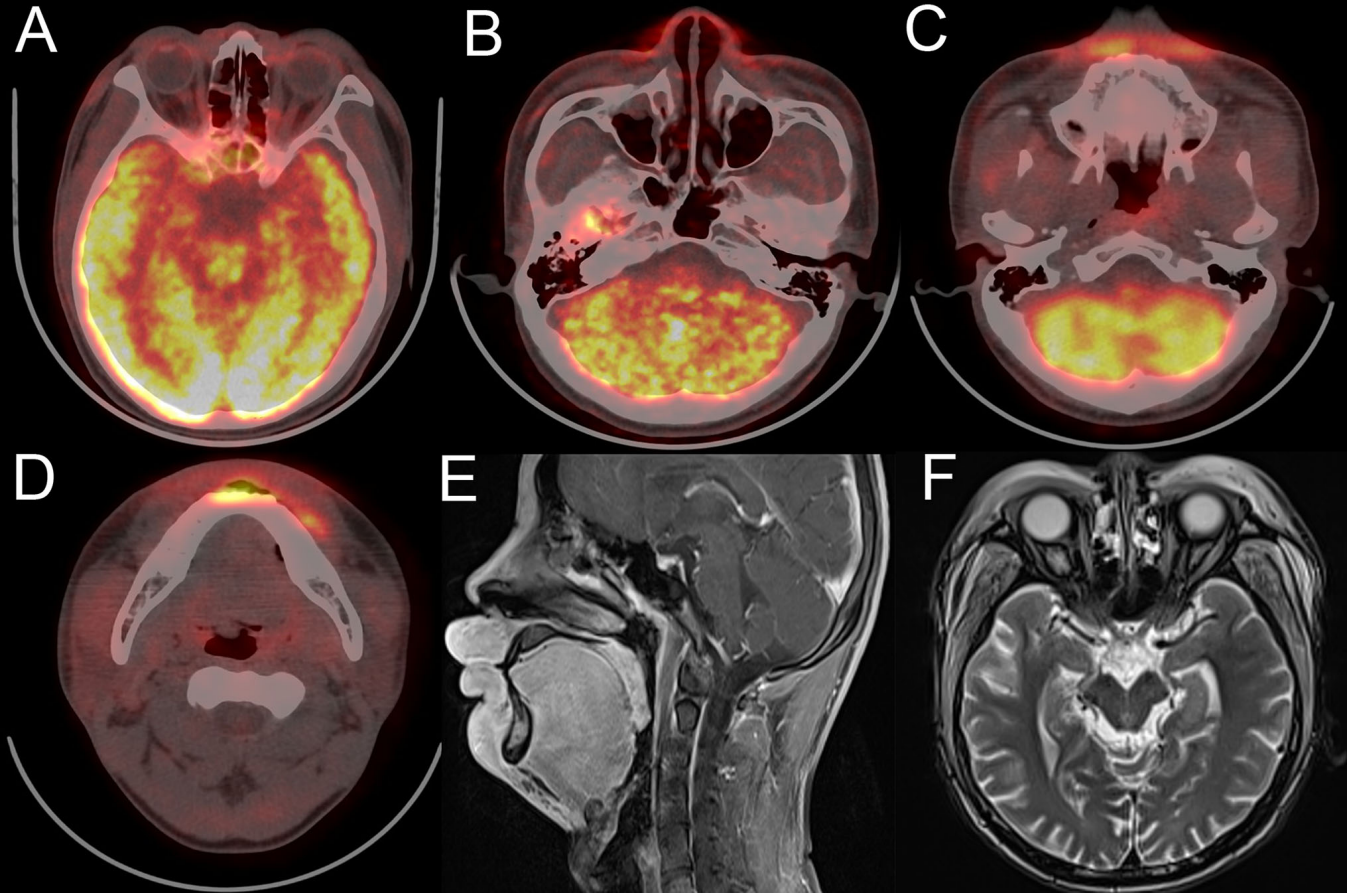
Face imaging features of the patient at diagnosis. (A–D) PET/CT shows thickened and FDG-avid midline facial skin. (E, F) MRI sagittal plane and horizontal views show thickened facial skin and hyperintensity on T2-weighted imaging.
